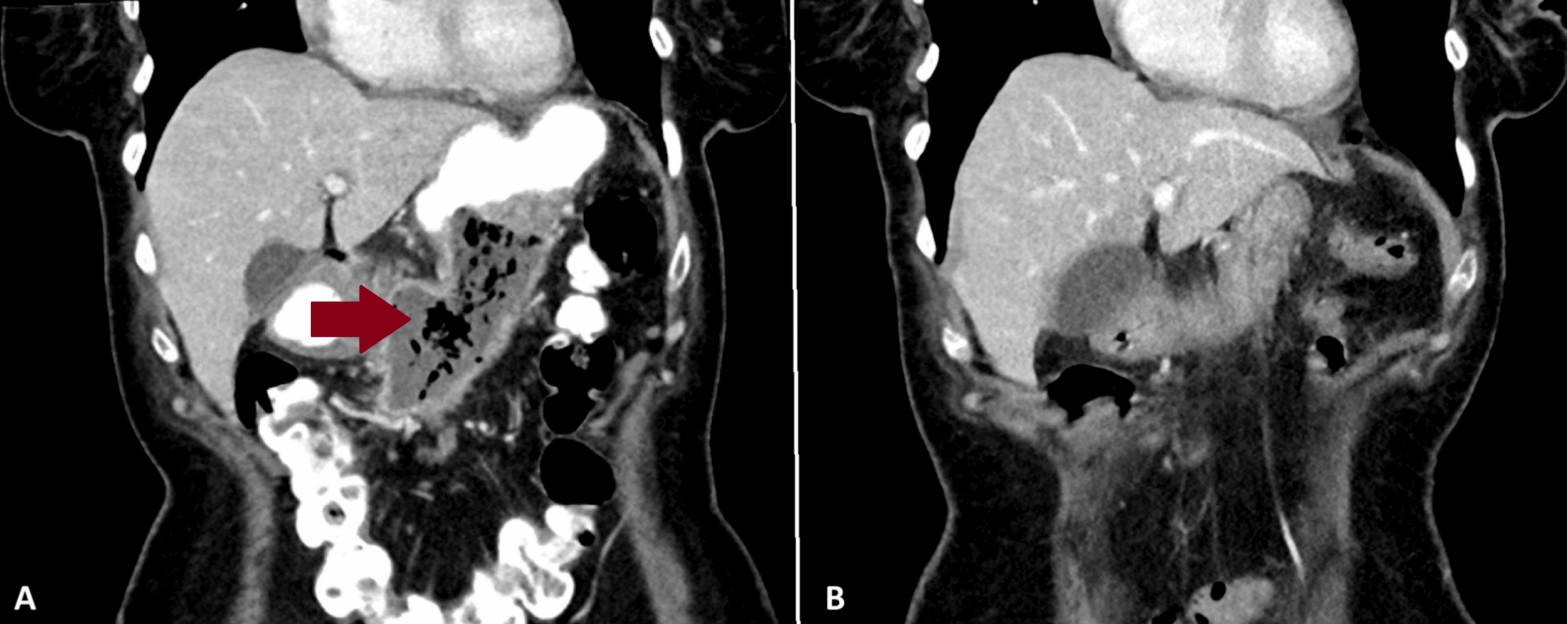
Abdominal CT images. (A) The initial abdominal CT with oral and IV contrast demonstrating peripancreatic necrosis and gas infiltration (arrow). (B) The control abdominal CT with IV contrast displaying no peripancreatic necrotic collections. CT: computed tomography; IV: intravenous.
